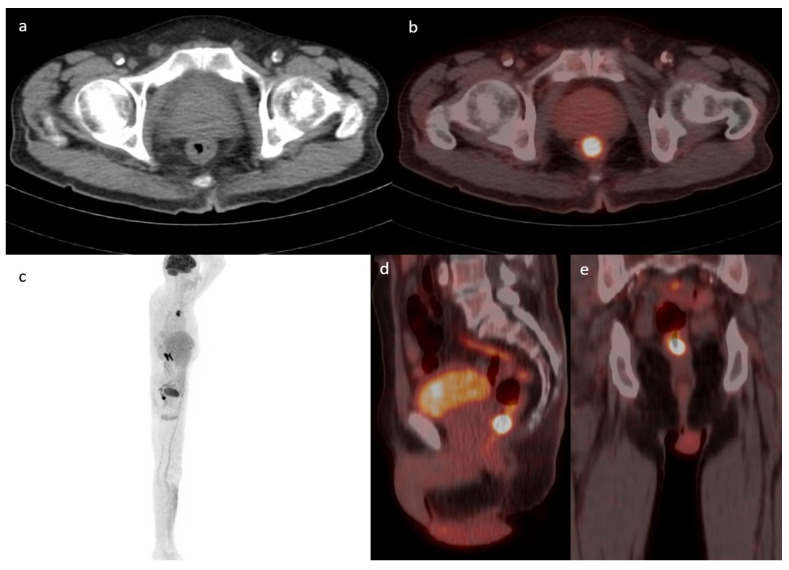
Case of a 71-year-old male known to have primary bladder cancer. Axial cuts of CT (a) fused with PET (b) showing a focus of increased uptake in the lower rectum (SUVmax 19.1) with corresponding circumferential wall thickening suspicious for malignancy. This lesion is redemonstrated in the MIP (c), sagittal (d), and coronal PET/CT cuts (e). Rectal biopsy confirmed the diagnosis of moderately differentiated adenocarcinoma.
